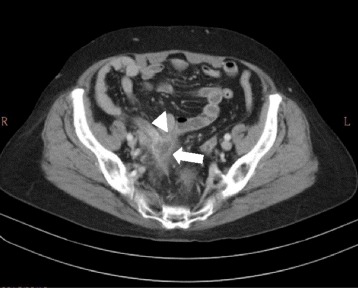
Contrast-enhanced pelvic computed tomography. The scan reveals one 2.1 × 1.6 cm irregular-shaped cystic mass (*arrow*) with marginal enhancement in the right pelvic cavity, abutting the swollen appendix (*arrowhead*). The tumor is located at the tip of appendix with possible subserosa invasion and attaches to the right pelvic side wall. The scan also shows inflammatory change with regional ileal bowel loop adhesion to the mass. Some fluid collection around the tumor-bowel complex is noted
